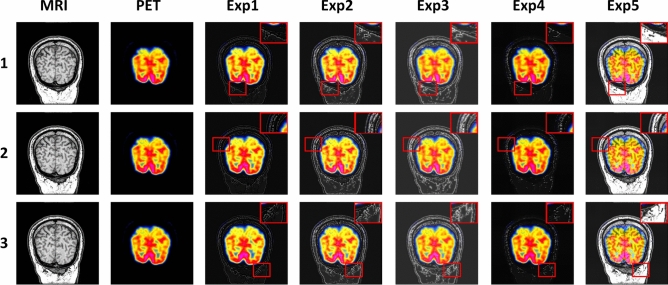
The ablation experiments fusion results of MRI brain images and PET images.
